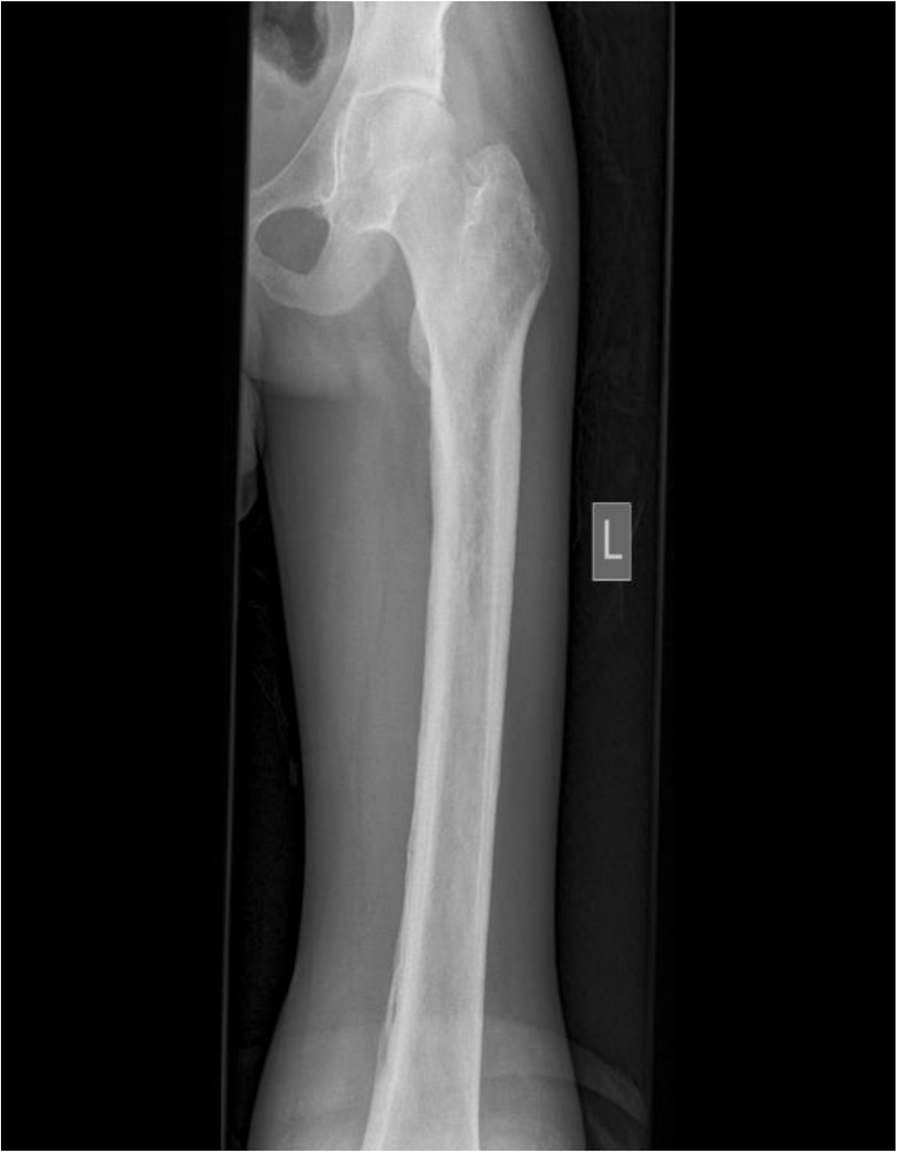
X-ray of femur showing periosteal thickening of medial cortex of femur
